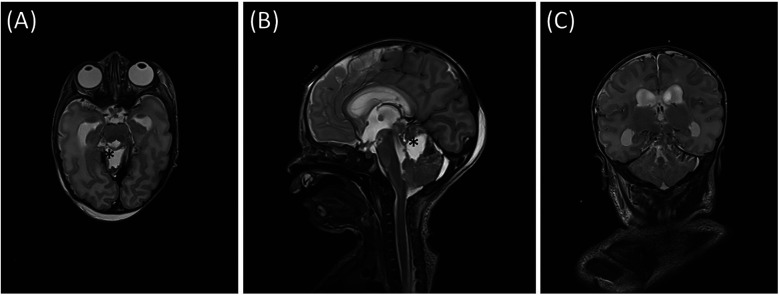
Corresponding planes of T2—MRI after surgery (A) axial, (B) sagittal, (C) coronal. * Hematoma cavity.
